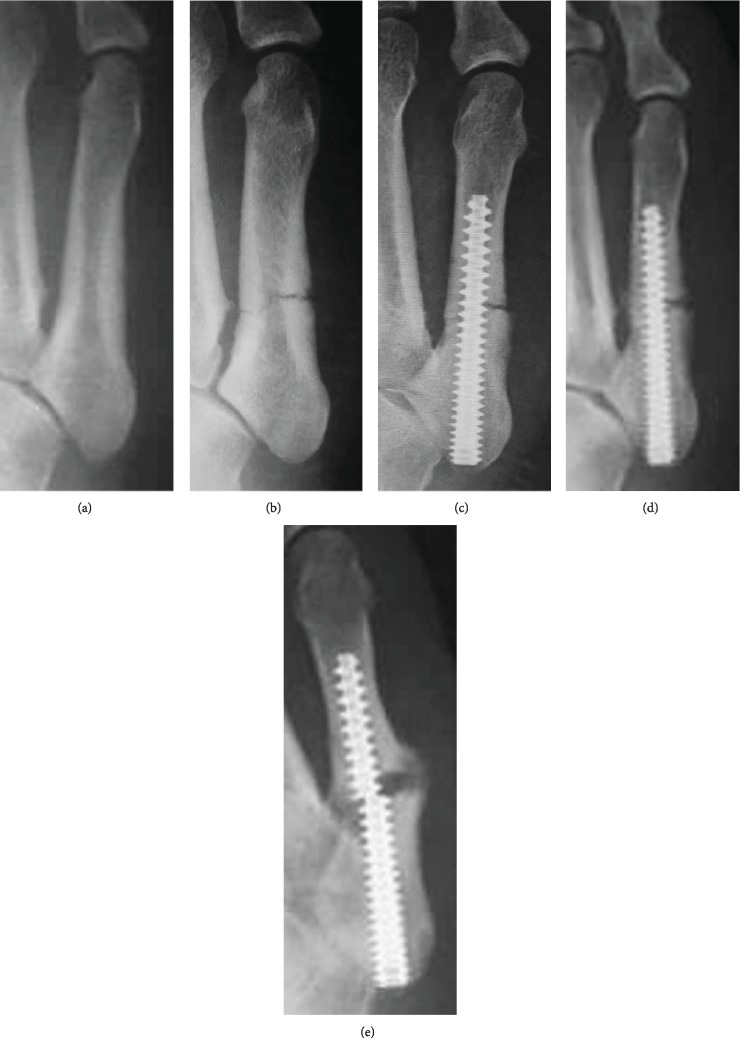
Case 3: anteroposterior radiographs of a 28-year-old sumo wrestler taken when he first visited another hospital (a) and 1 week later (b). We treated the Jones fracture operatively at 2 weeks after the first visit (c). Radiographs taken 4 weeks postoperatively are shown (d). At 4 months after operation, a screw broke (e).
